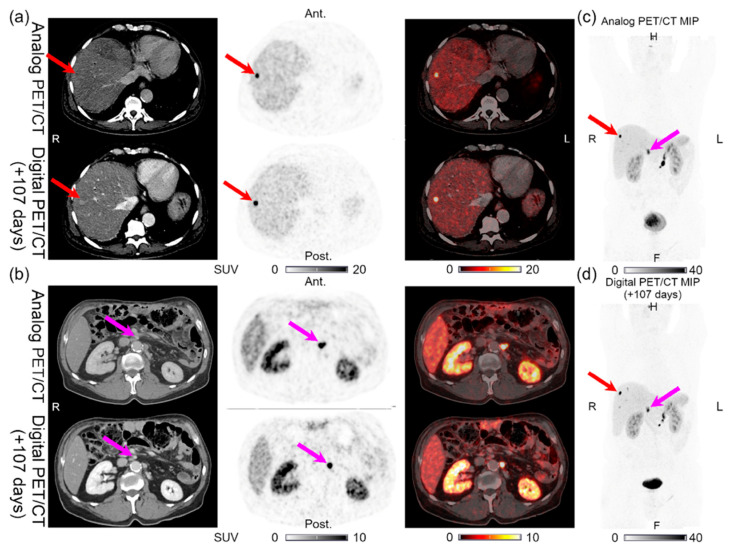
Patient 3: (a) liver lesion and (b) lymph node lesion with structural CT correlates found on both the analog PET and the digital PET performed 107 days later. (c) MIP for the analog PET and (d) MIP for the digital PET with arrows showing the lesion location.
